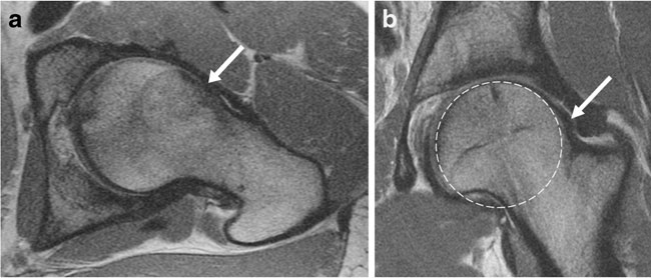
Oblique axial (a) and coronal (b) proton density (PD)-weighted MRI images in a 30-year-old man with a cam deformity. There is an osseous protuberance (*arrows*) at the femoral head–neck junction anterolaterally, with loss of offset of the femoral head–neck junction. The physeal scar extends lateral to the circular region of the femoral head (*dashed circle*) on the coronal image
